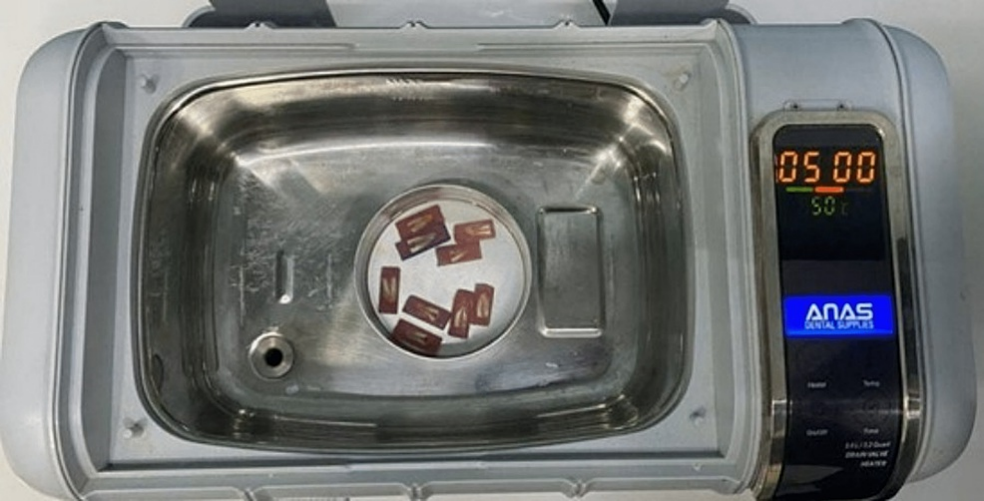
The samples inside the ultrasonic vibrator.
